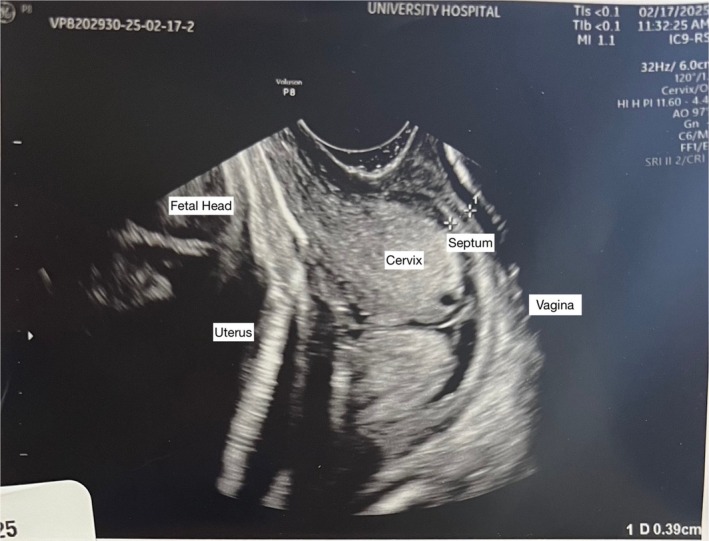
Annotated point‐of‐care transvaginal ultrasound image demonstrating transverse vaginal septum at time of diagnosis.
